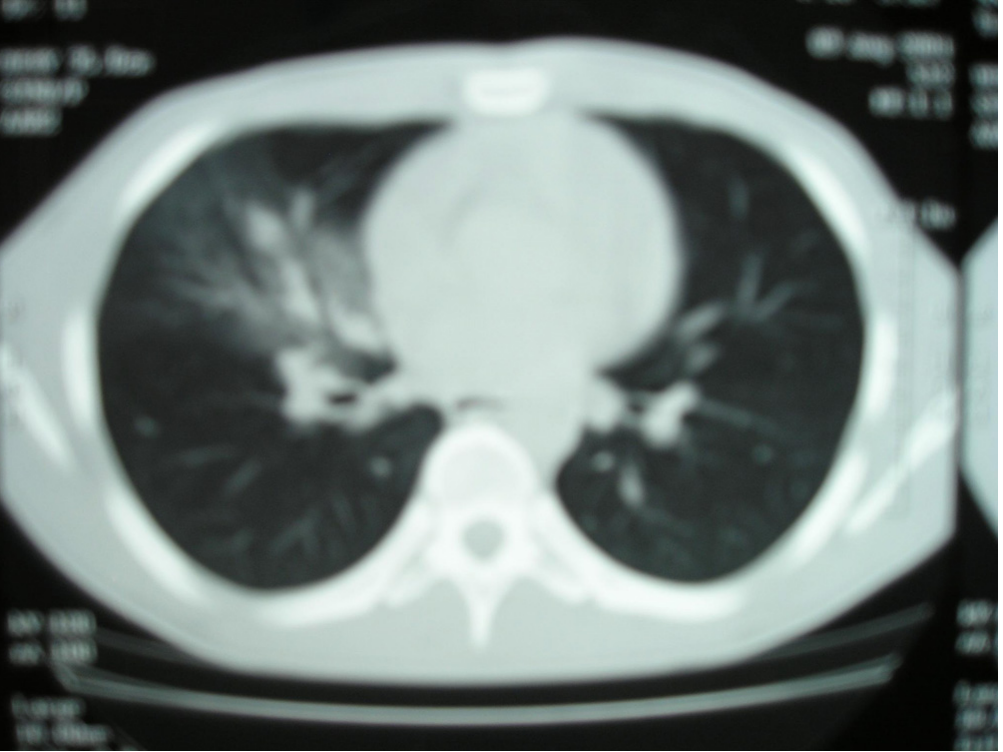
Computed tomography of the chest following 6 days of treatment showing significant improvement.
